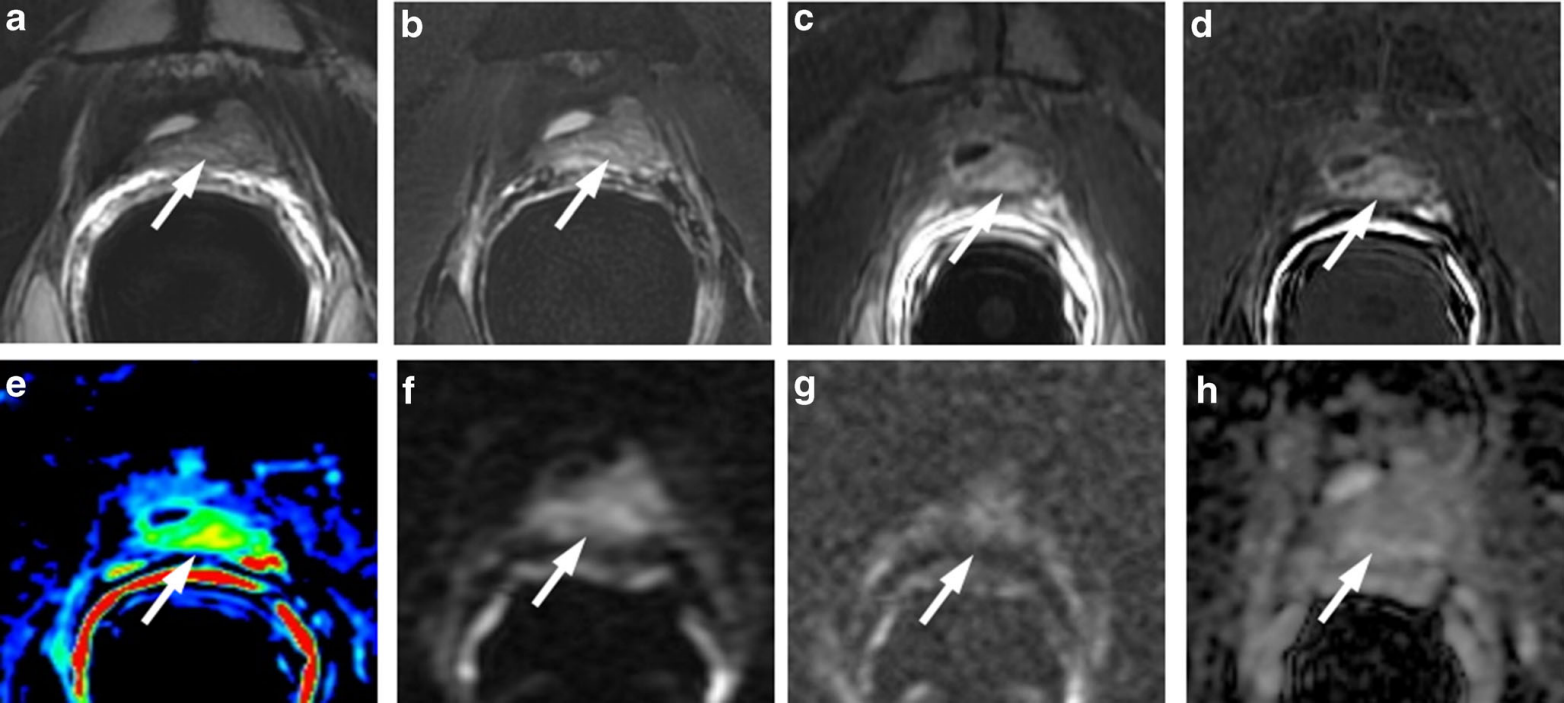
MR images of a 69-year-old man with prostate-specific antigen progression (PSA serum level 1.06 ng/mL) after radical retropubic prostatectomy, with suspected local recurrence. a Axial T2-weighted fast spin-echo (5525/109) and b axial T2-weighted fat saturated fast spin-echo image (5981/116) show a solid tissue on posterior perianastomotic location in front of the rectal wall at about 33 mm from the ureteral meatus which is hyperintense compared to pelvic muscles (*white arrows*). c Axial gradient-echo T1-weighted, d gradient-echo T1-weighted subtracted image and e DCE MR colour map showing a positive contrast enhancement of the abnormal tissue. f Diffusion weighted images with a b value of 1.000,g 3.000 s/mm^2^ and h ADC map show no diffusion restricted phenomena, consistent with residual glandular tissue
